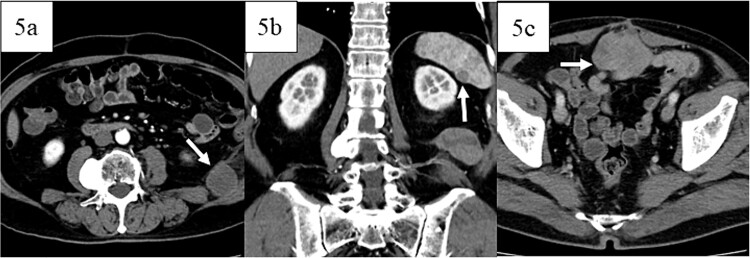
Images of contrast-enhanced computed tomography, contrast-enhanced computed tomography scan revealed recurrence in the retroperitoneum 12 months postoperatively (white arrow in 5a) and metastases in the spleen 16 months postoperatively (white arrow in 5b), and peritoneal dissemination (white arrow in 5c) 27 months postoperatively.
